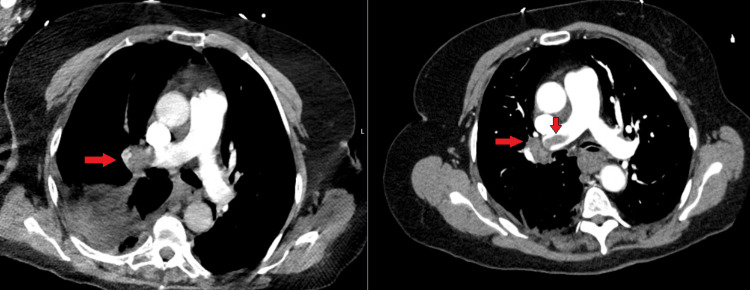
Pulmonary embolism on computed tomography angiography (CTA) chest CTA Chest (mediastinal windows) demonstrating right main pulmonary embolism (red arrows) in the emergency department (left) vs initial hospitalization (right).
